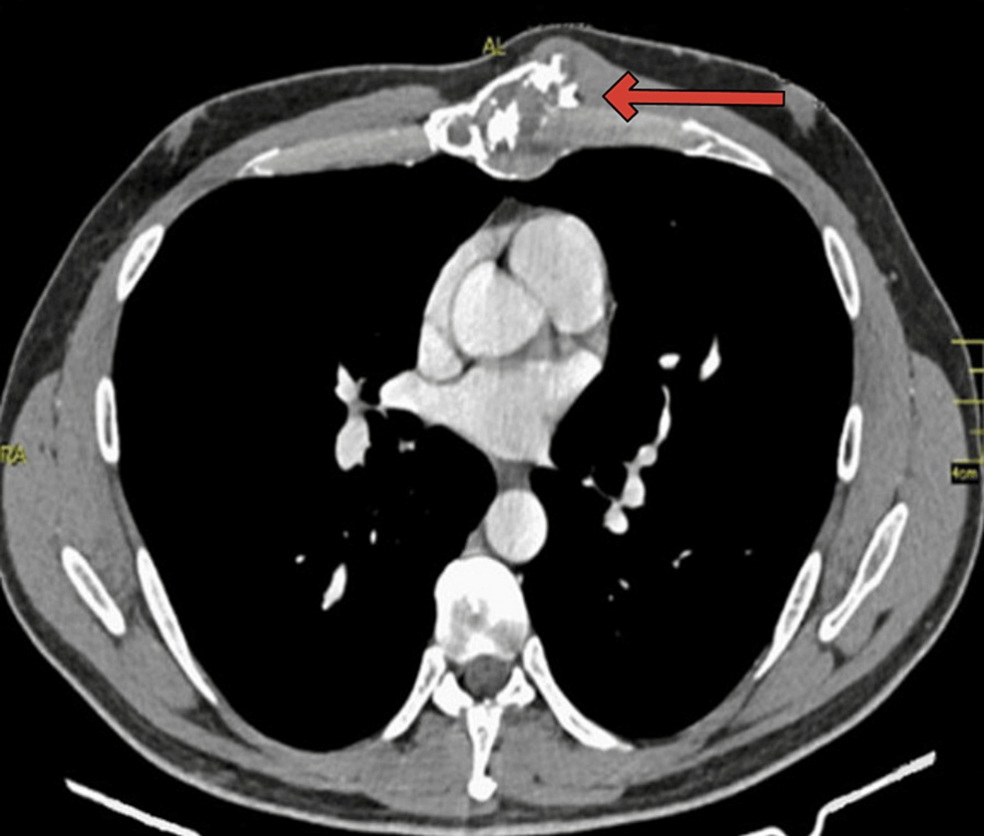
CT scan of the thorax revealing an expansive process in the sternum The chest CT image in a soft tissue window setting shows an expansive process in the sternum with coarse mineralization, indicated by a red arrow.
